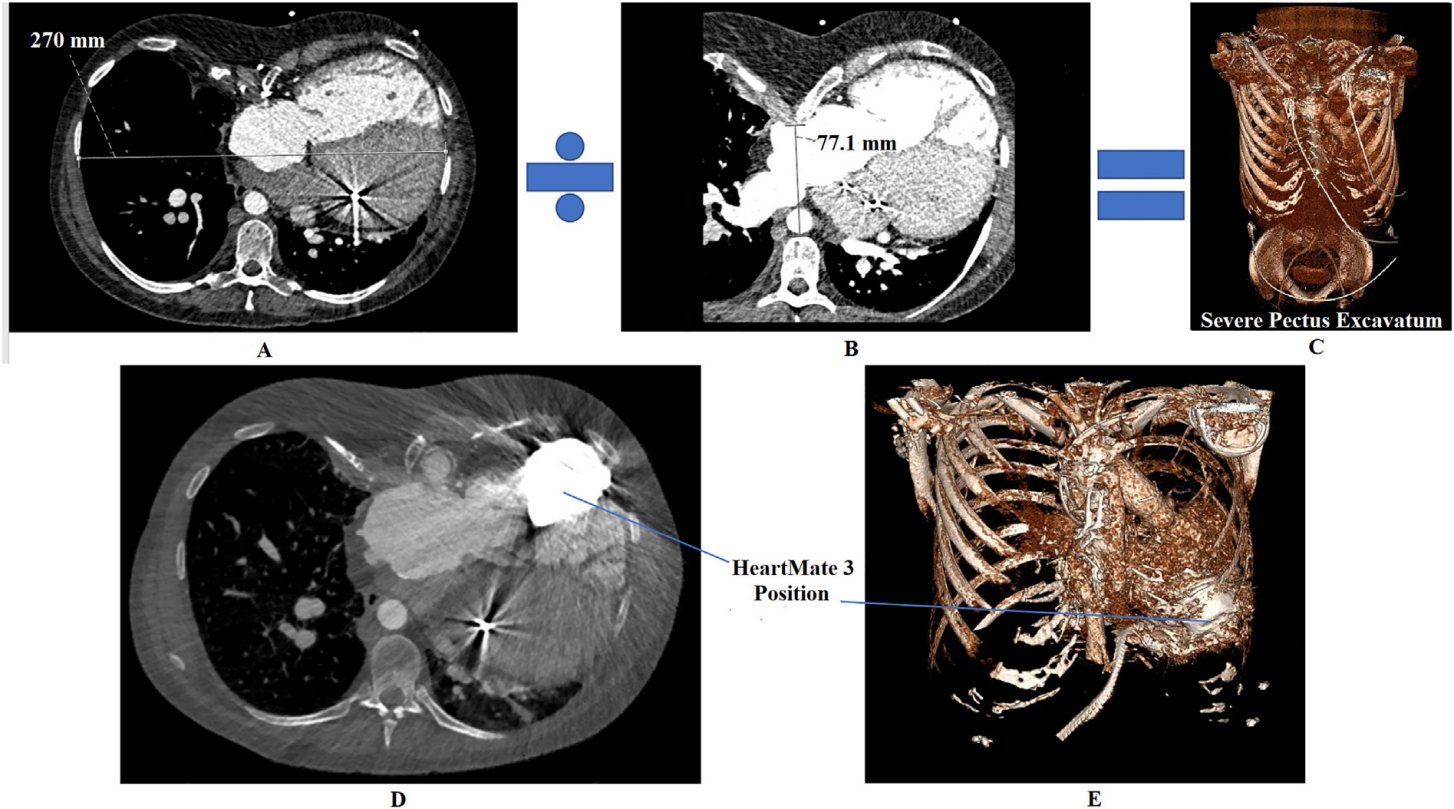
Pre- and post-procedural chest computed tomography (CT) scan. Pre-procedural chest CT scan: Calculation of Haller index in our patient by dividing the (A) transverse diameter of the chest by the (B) distance between the anterior angle of the spine and the posterior aspect of the deepest part of the sternum. Panel C shows the 3-dimensional image of the pectus excavatum in our patient. Postprocedural chest CT scan: Panel D shows position of the HeartMate 3 with respect to the sternum and the right ventricle and the 3-dimensional image for better demonstration is shown in panel E.
